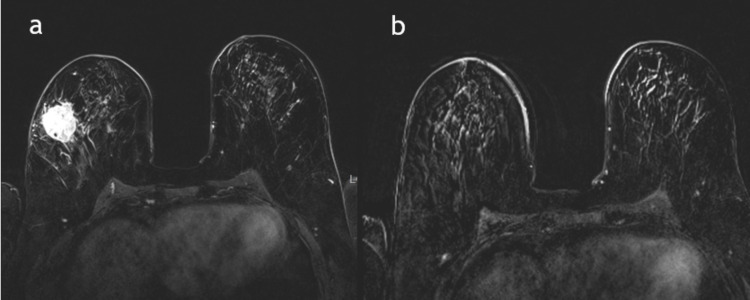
MRI pre and post-NAC. A 52-year-old female patient with right-sided invasive ductal carcinoma; (a) postcontrast MRI image before the start of NAC shows a well-defined enhancing right breast mass, and (b) postcontrast MRI after NAC shows complete resolution. MRI: magnetic resonance imaging; NAC: neoadjuvant chemotherapy
